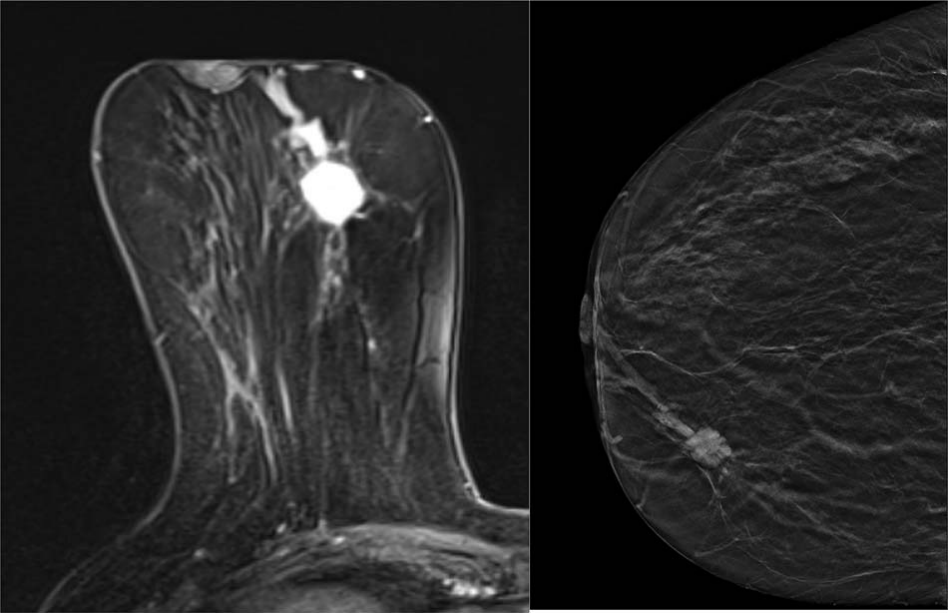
MRI of Rt breast (left), Tomosynthesis of Rt breast (right).
